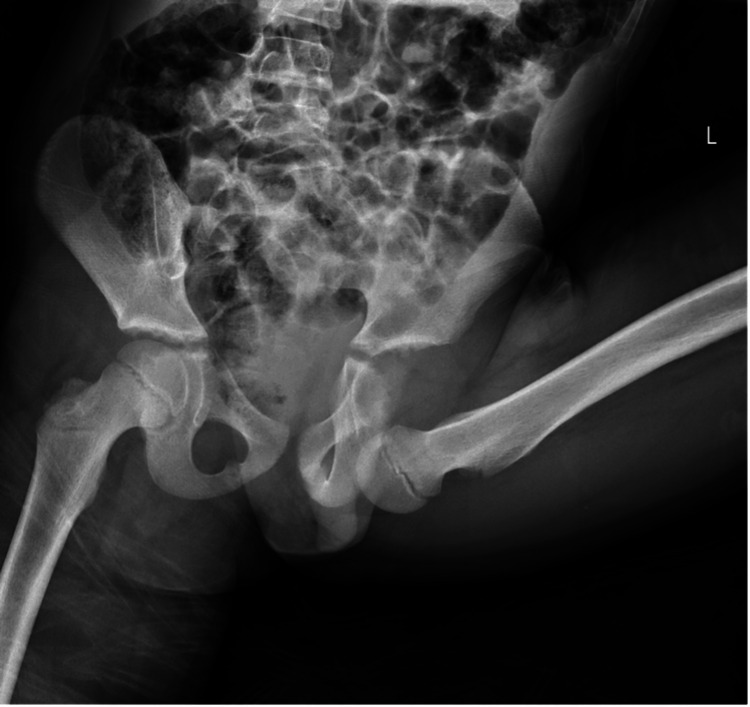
X-ray of the child demonstrating an anterior obturator type dislocation of the left hip.
